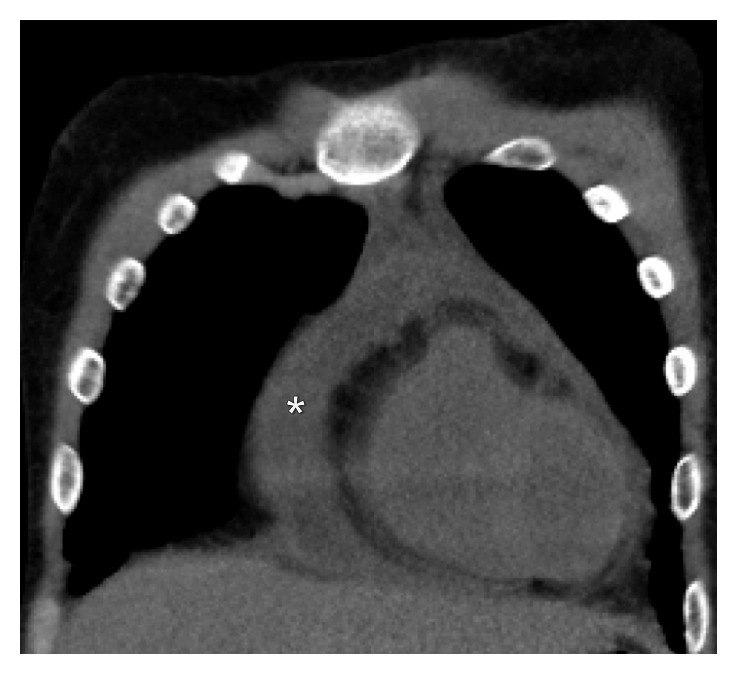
CT chest coronal view (pericardial effusion denoted by ∗).
